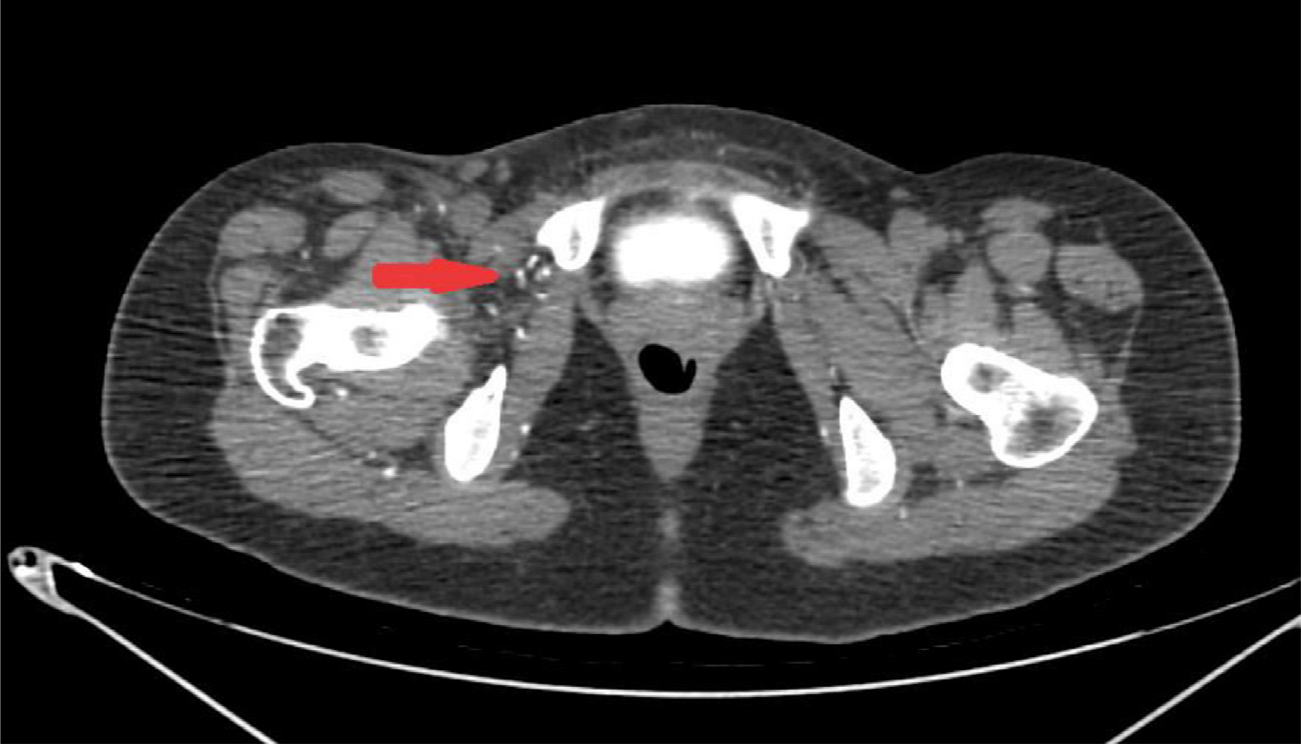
Axial abdominopelvic CT scan with contrast: There are several collateral vessels on the right side of the abdominal wall and extraperitoneal (arrow).
